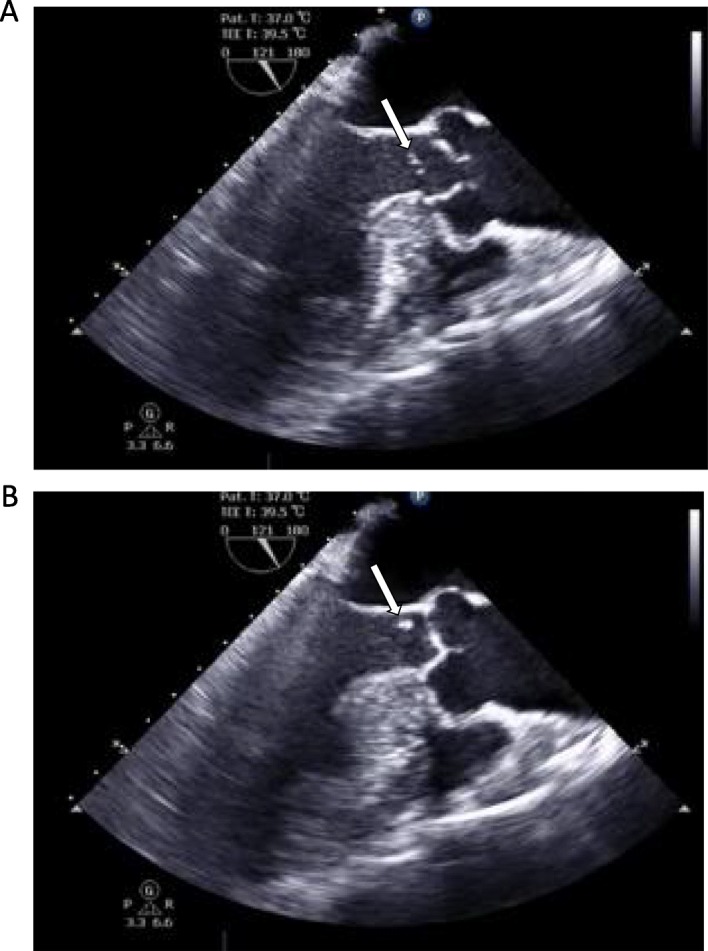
A subaortic floating object detected by transesophageal echocardiography. A subaortic membranous object (white arrow) moves up and down with a cardiac cycle. 1a, systolic phase; 1b, diastolic phase
